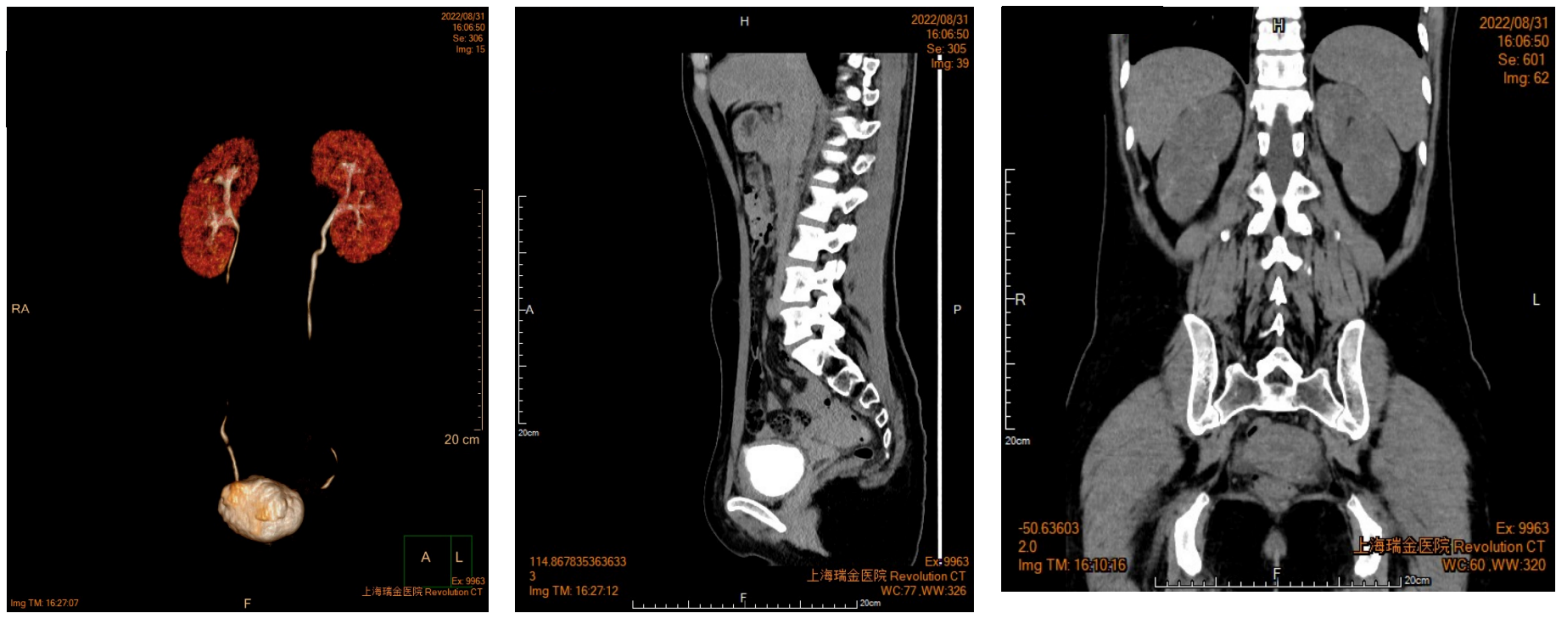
Enhanced CT of urinary system indicated multiple strips of slightly high density in both kidneys.
